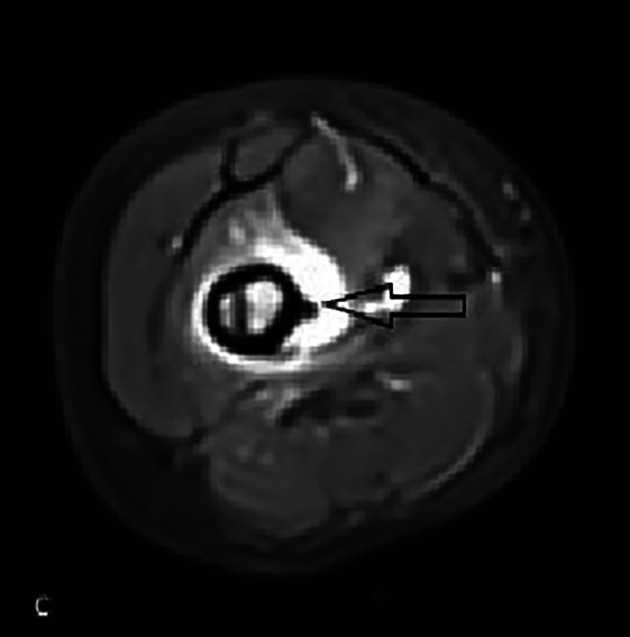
A Fat‑saturated PD‑WI MRI. Increased signal in the femoral diaphyses. B–C Fat‑saturated T1‑WI MRI with contrast. Subperiosteal collection with peripheral rim enhancement (arrow).
